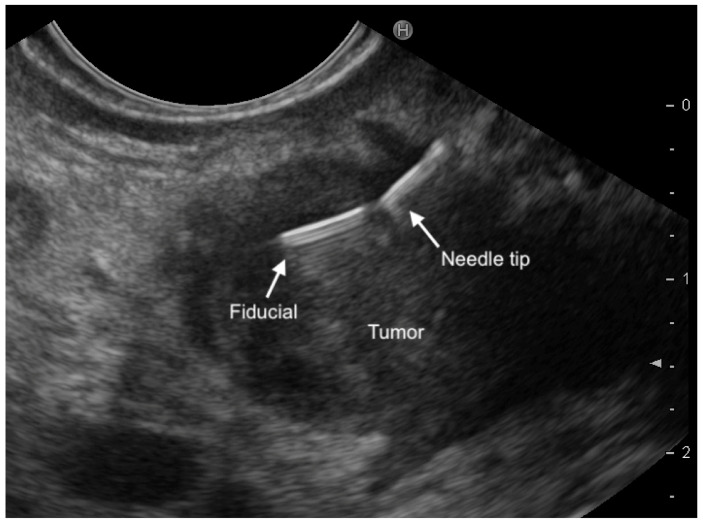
A magnified endoscopic ultrasound view demonstrating a fiducial just released inside a small pancreatic lesion.
